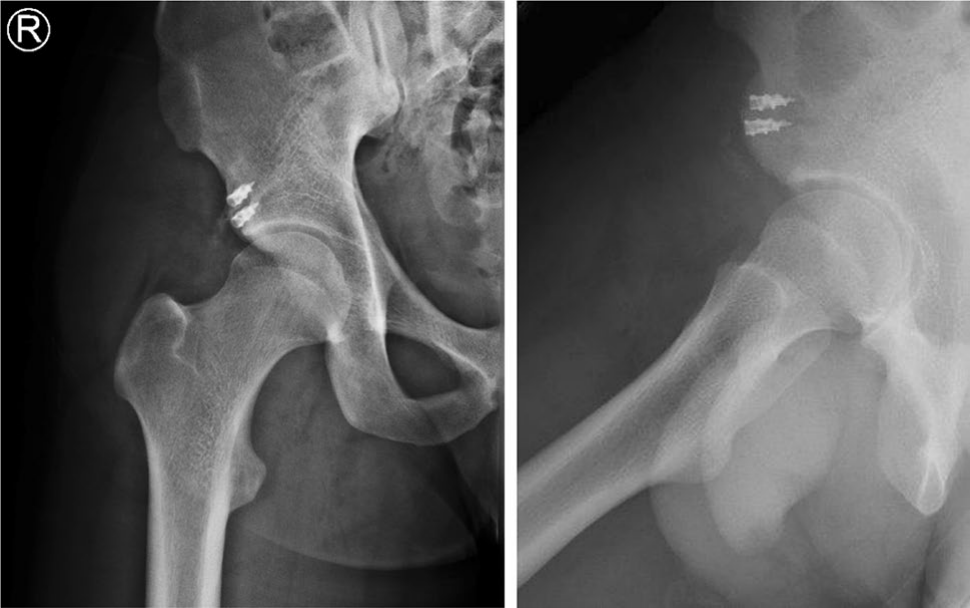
Postoperative radiographs. The antero-posterior and false-profile X-rays of the right hip show correct anchor placement at the anterior inferior iliac spine (straight head was reduced to superior anchor) and the superior acetabular ridge (reflected head was reduced to inferior anchor) with two double-loaded 5.5-mm titanium suture anchors (Corkscrew®, Arthrex, Naples, USA)
